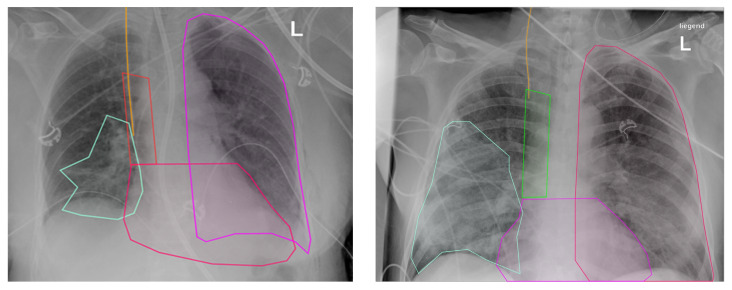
Annotated chest radiographs.
